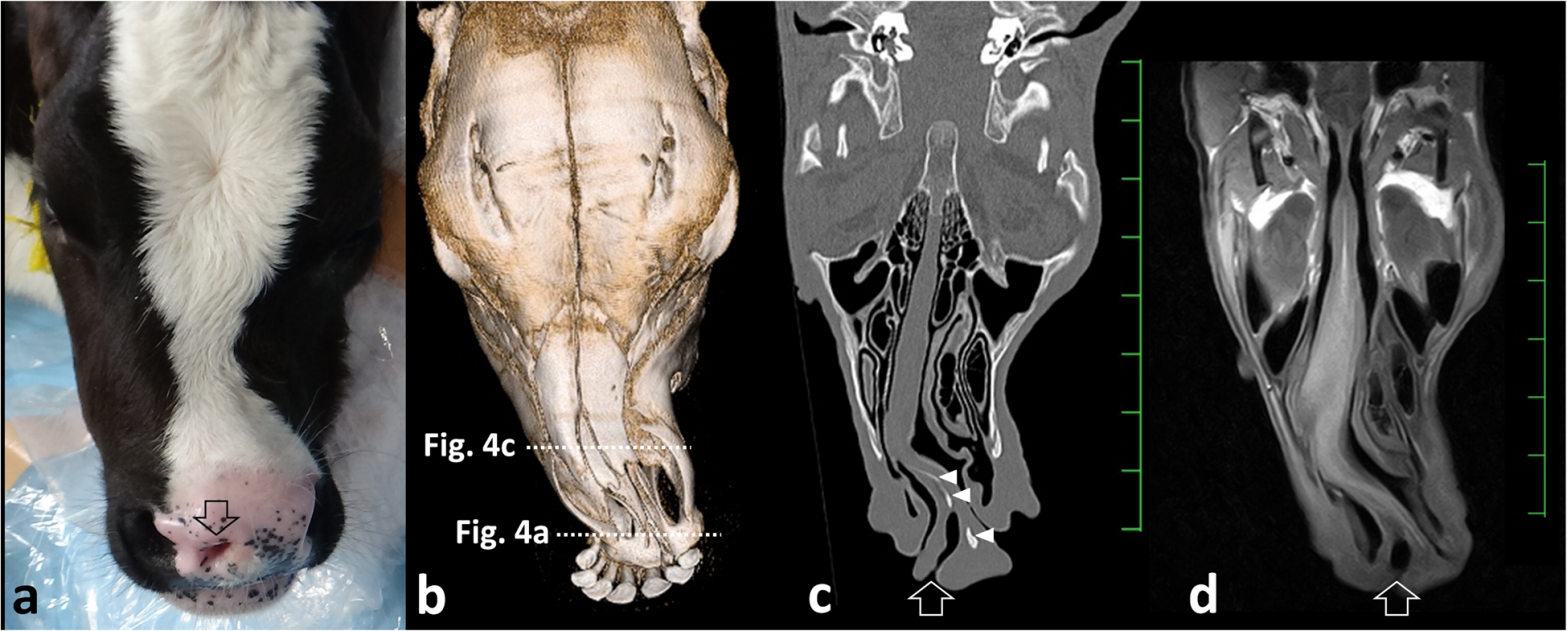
Dorsal macroscopic view of the nose (a), three-dimensional computed tomography (CT) visualizing the dorsal surface of the nasal bones (b), and dorsal CT (c) and T1-weighted MRI (d) visualizing the nasal cavity in the 1-month-old female Holstein calf. a The bridge of the nose is significantly curved toward the left. The middle nostril (empty arrow) is located in close proximity to the right nostril. b The transformation of the nasal bone allowed a curvature of approximately 30° from the central line of the nasal bone to the left. c The abnormal curve of the nasal septum allowed constriction within the right nasal cavity. The blind end is evident within the middle nostril (empty arrow). Bone-like structures (white arrowheads) are observed within the nasal septum. d A blind-ended middle nostril is evident (empty arrow), although a bone-like structure is not evident. Scale: 25 mm on CT and MRI
